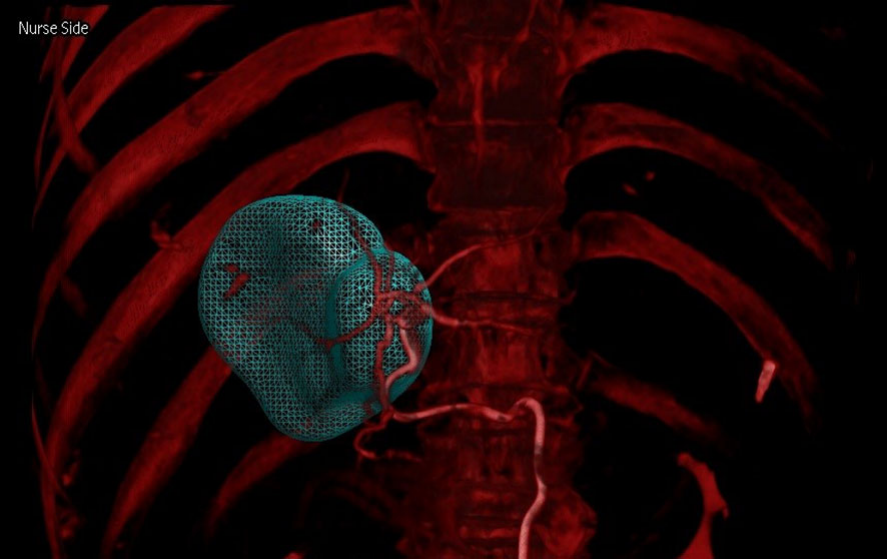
Combining arterial and venous phase CBCT imaging (dual CBCT-overlay image) enables rapid and accurate identification of tumor-supplying arteries, facilitating more precise ^90^Y therapy delivery.
